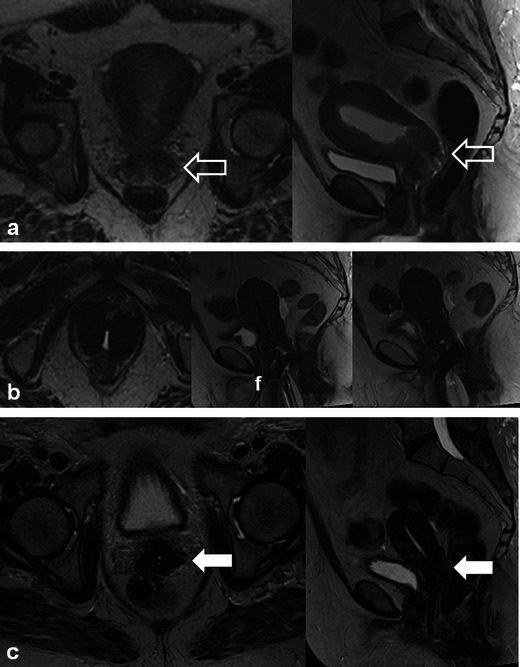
A 60-year-old woman with cervical squamous cell carcinoma, treated with brachytherapy, demonstrating complete response to treatment. Initial staging pelvic MRI depicts the fungating cervical mass with left parametrial invasion (*open white arrows*) on coronal oblique and sagittal T2 TSE (a). Also note the mass is causing malignant hydrometra due to cervical obstruction. Follow-up pelvic MRI for treatment planning depicts optimal positioning of ovoid and tandem intracavitary brachytherapy device on axial and sagittal T2 TSE with intracavitary device at the level of the cervical tumour and probe tip in the endometrial cavity. Foley catheter (f) in situ. Follow-up pelvic MRI performed 1 year after RT demonstrates complete response with reconstitution of the normal zonal anatomy of the cervix and presence of homogeneous low signal intensity of the cervical stroma (*white arrows*) on axial and sagittal T2 TSE (c), which are reliable indicators of complete response and absence of tumour in the post-irradiation cervix. Low T2 signal has replaced intermediate signal in the left parametrium (*white arrow* on axial image in c) indicating parametrial fibrosis
